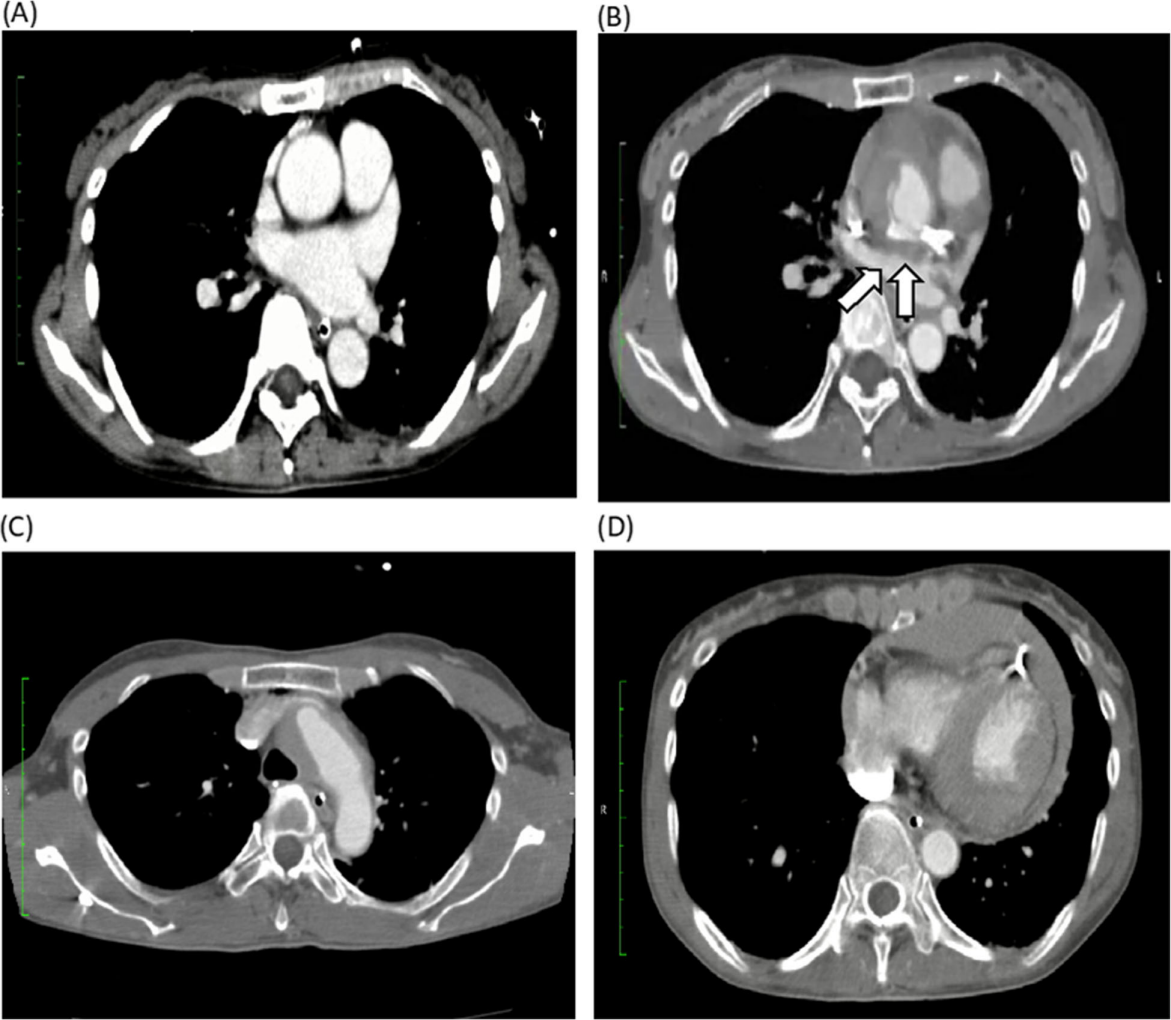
Computed tomography of the patient’s chest. a, Before percutanous coronary intervention (PCI), no aortic dissection was observed. b, After PCI, a new dissection appeared in the ascending aorta (arrow). The false lumen is non-communicating. c, Dissected aortic arch. d, A massive bloody pericardial fluid appeared, which was not present before PCI
